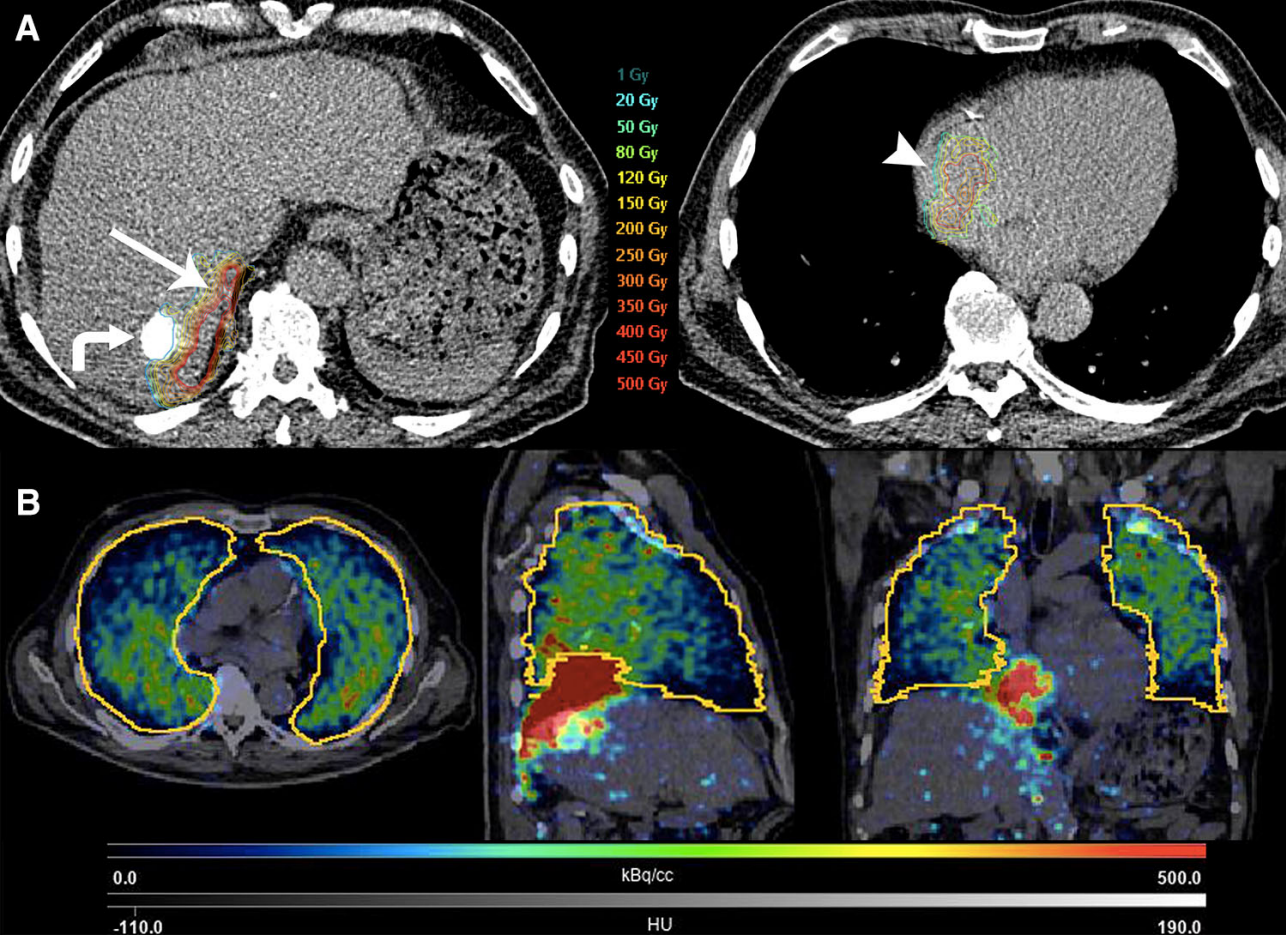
A Post-treatment ^90^Y-PET-CT dose distribution (Simplicit^90^Y/BTG) to the atrial tumor (arrowhead) and adjacent diaphragm (arrow). Previously treated HCC lesion filled with Lipiodol (curved arrow). B Post-treatment ^90^Y-PET-CT multiplanar dose distribution (PMOD Technologies) in the lungs with a gradient, predominant in the posterior parts
